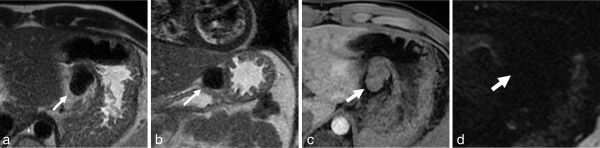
Findings on MRI. Arrows show the mass at the lesser curvature. (a, b) The mass shows a dark intensity on *T*_2_ weighted imaging. (c) The mass shows signal intensity almost equal to that of the gastric wall on *T*_1_ weighted imaging, but contains an area of darker intensity. This area was consistent with the calcification seen on the CT scan, so it was considered to indicate calcification. (d) The mass does not show restricted diffusion.
